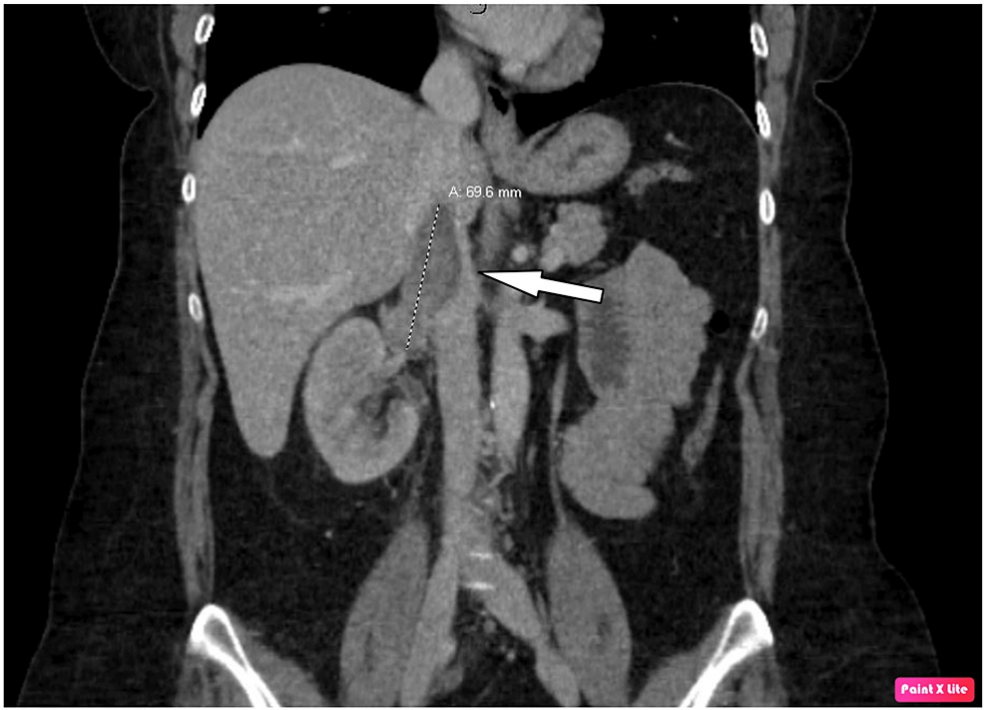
CT of the abdomen and pelvis showing a right renal mass with inferior vena cava extension (white arrow) CT: computed tomography
